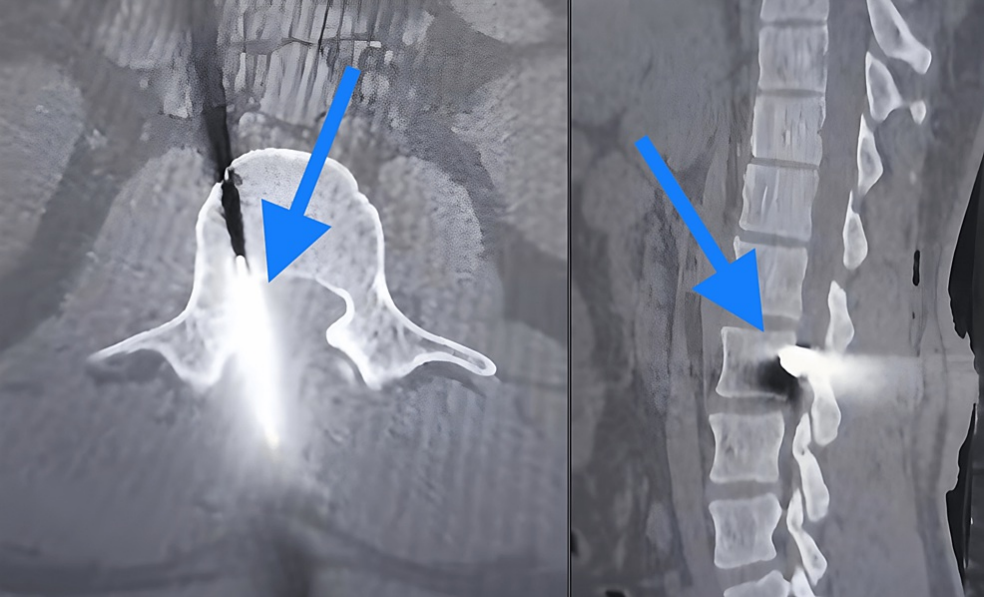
CT scan images Axial and sagittal CT cuts showing knife blade extending into the vertebral canal of the L2 vertebra
